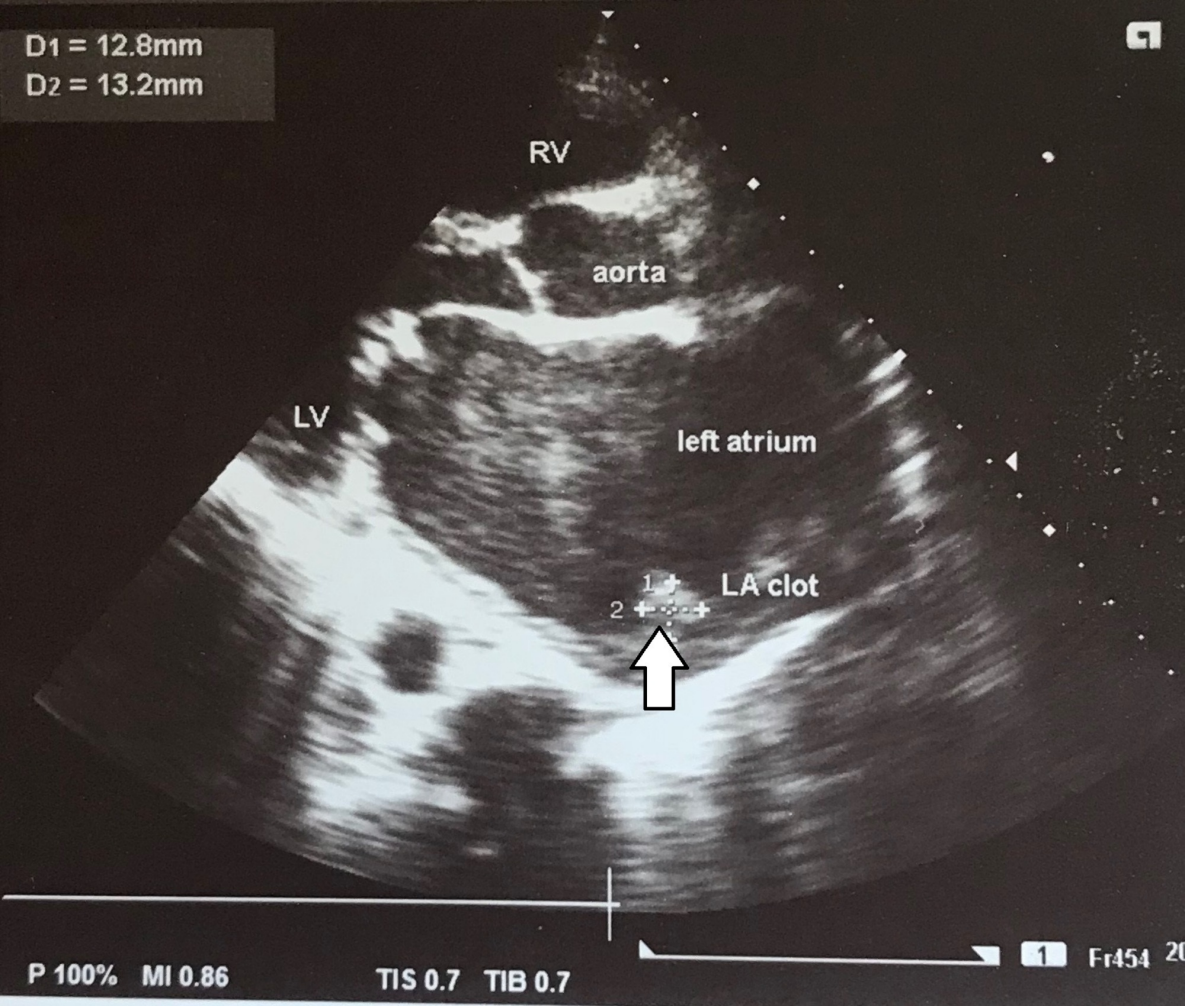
Transthoracic echocardiography on parasternal long axis view showing left atrial enlargement, left atrial clot measuring 12.8 mm x 13.2 mm (arrow), and thickened and calcified mitral valve LV: left ventricle; LA: left atrium; RV: right ventricle; D: diameter.
